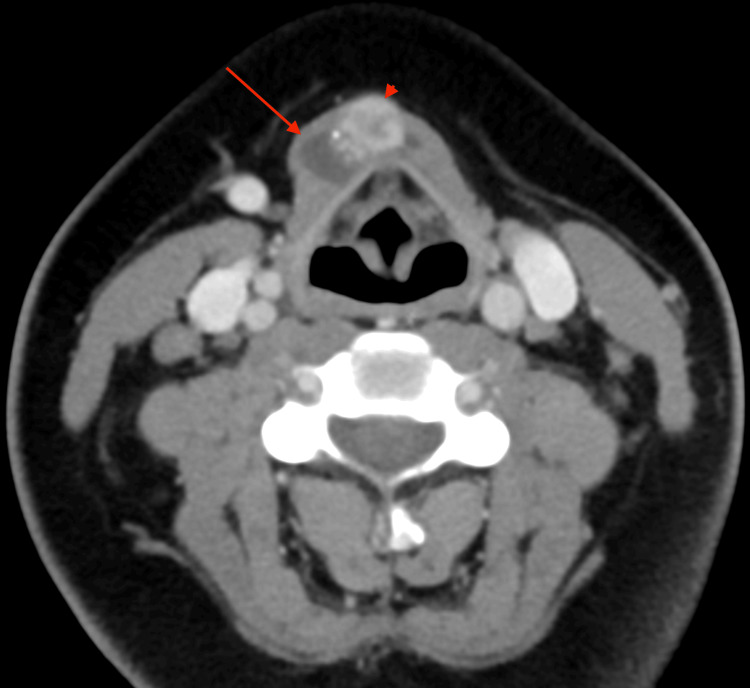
Axial CT of the neck with contrast. Axial CT revealed a cystic tubular structure slightly to the right of the midline (red arrow). There was a peripherally hyperattenuating and centrally hypoattenuating lesion (red arrowhead) in the superior midline aspect of the cyst with multiple adjacent punctate calcifications, measuring 1.0 x 1.2 x 1.3 cm.
